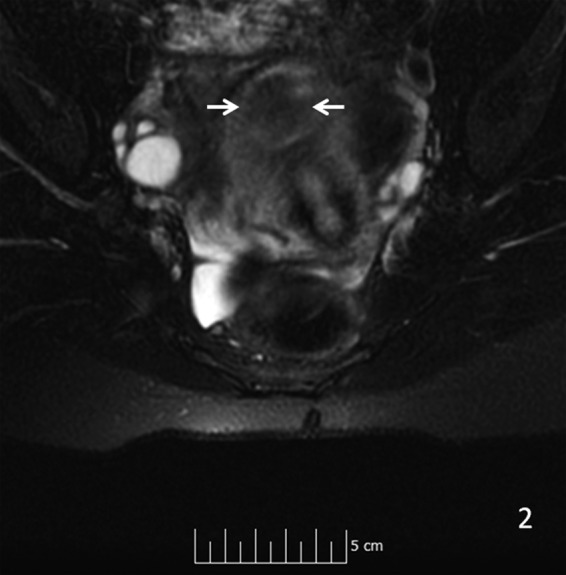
Axial T2 MRI shows arrows pointing to an intermediate signal strength in the mass.
